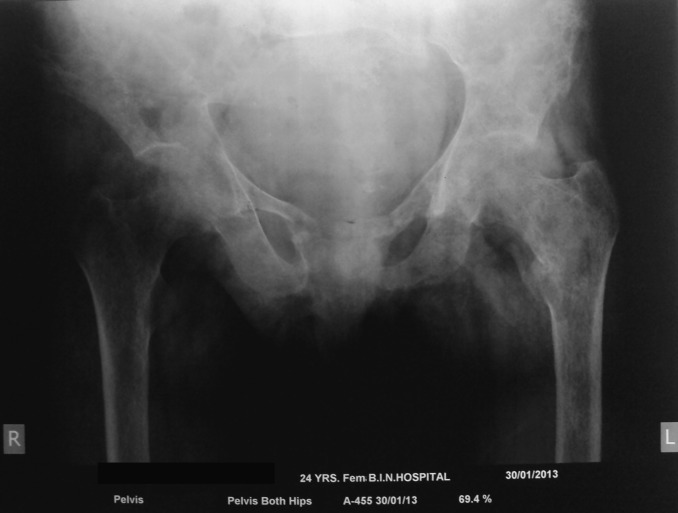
X-ray of the pelvis showing multiple extensive lytic lesions involving the iliac bones, pubic rami, and greater trochanters of the femur along with cortical thinning and increased lucency of the bones consistent with osteitis fibrosa cystica (Von Recklinghausen's disease of bone).
